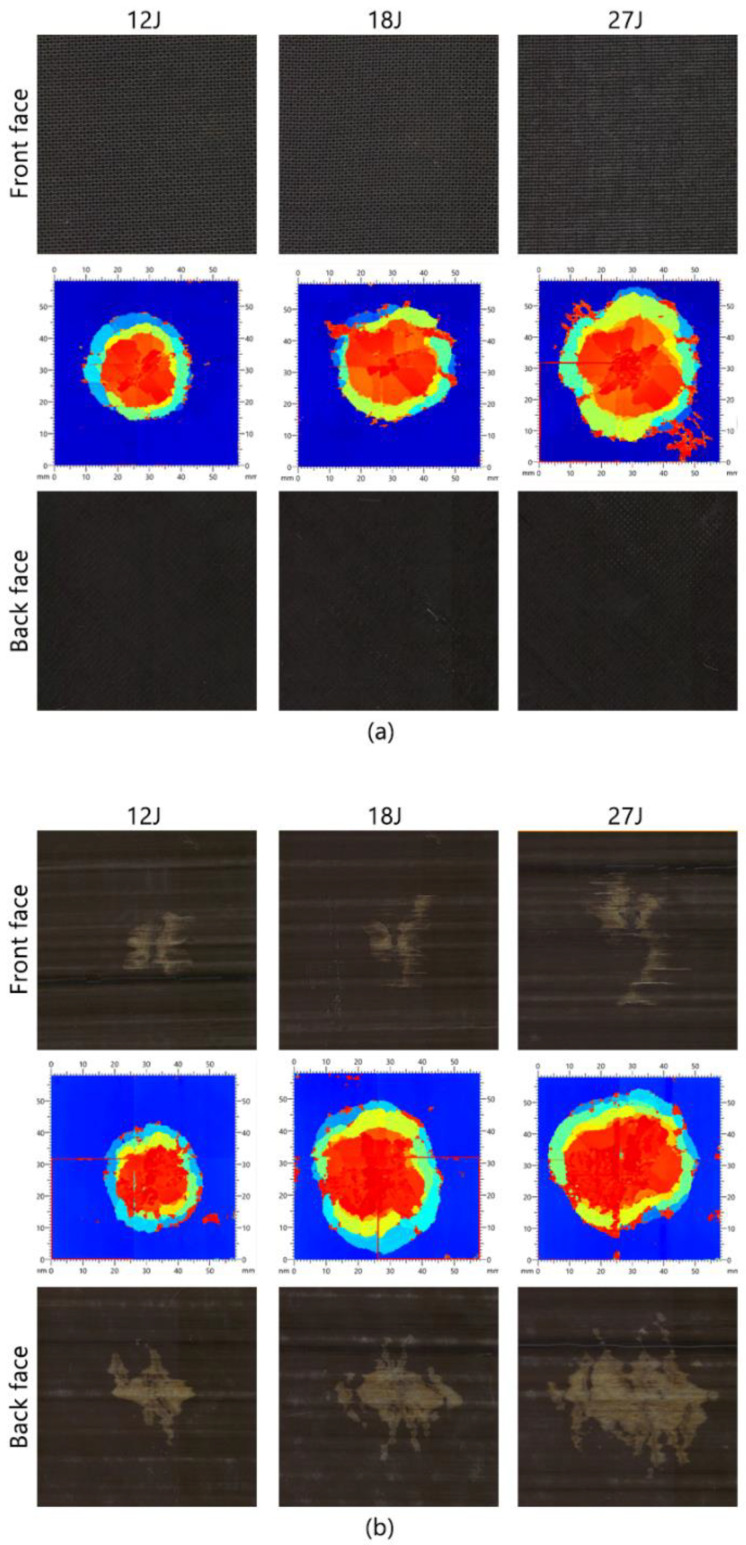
Ultrasonic C-scan and visual inspection results: (a) *reference*; (b) *sensor*.
